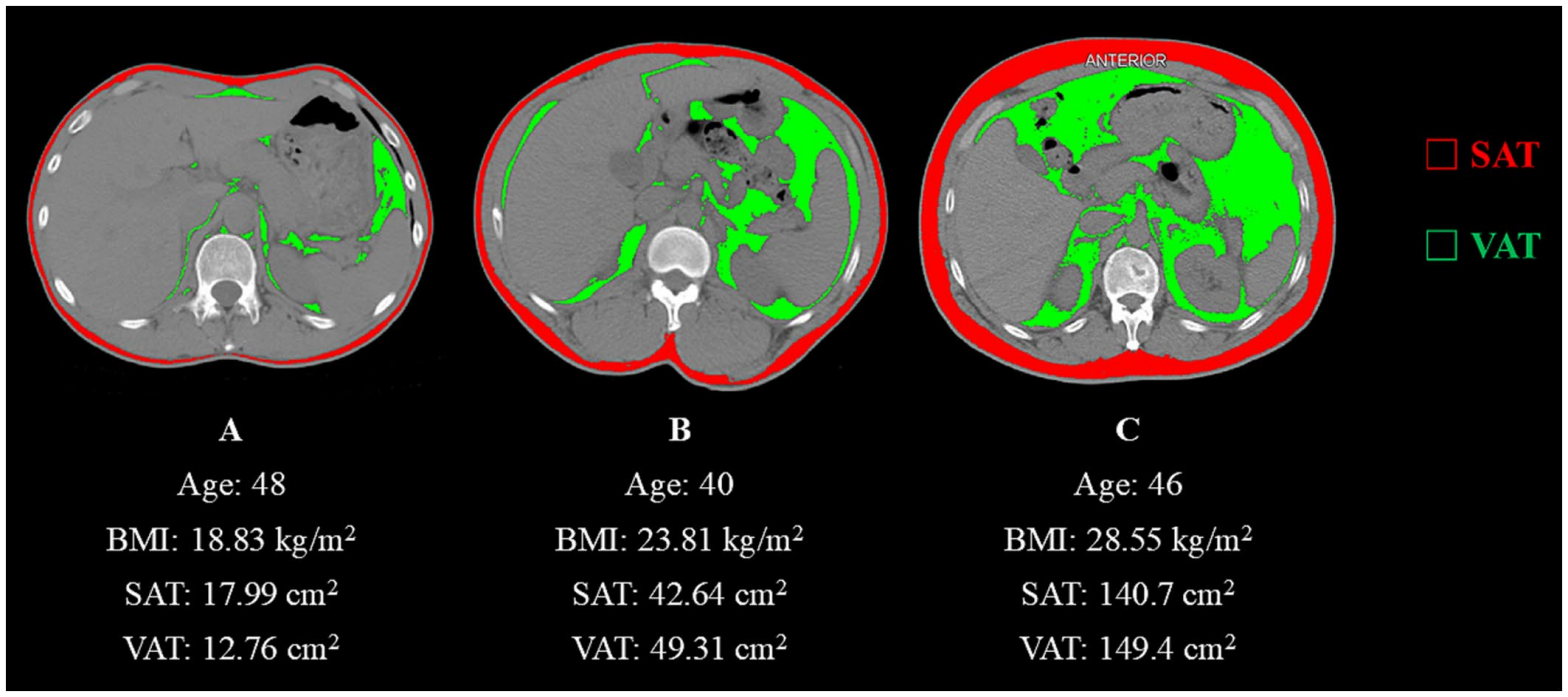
(A) Abdominal CT image with a Hounsfield unit-based color scale of adipose area in a 48-year-old man with a smoking history shows a lean physique. (B) Abdominal CT image with a Hounsfield unit-based color scale of adipose area in a 40-year-old man with a history of occupational paint exposure displays intermediate adiposity. (C) Abdominal CT image with a Hounsfield unit-based color scale of adipose area in a 40-year-old manifests marked obesity-related characteristics.
